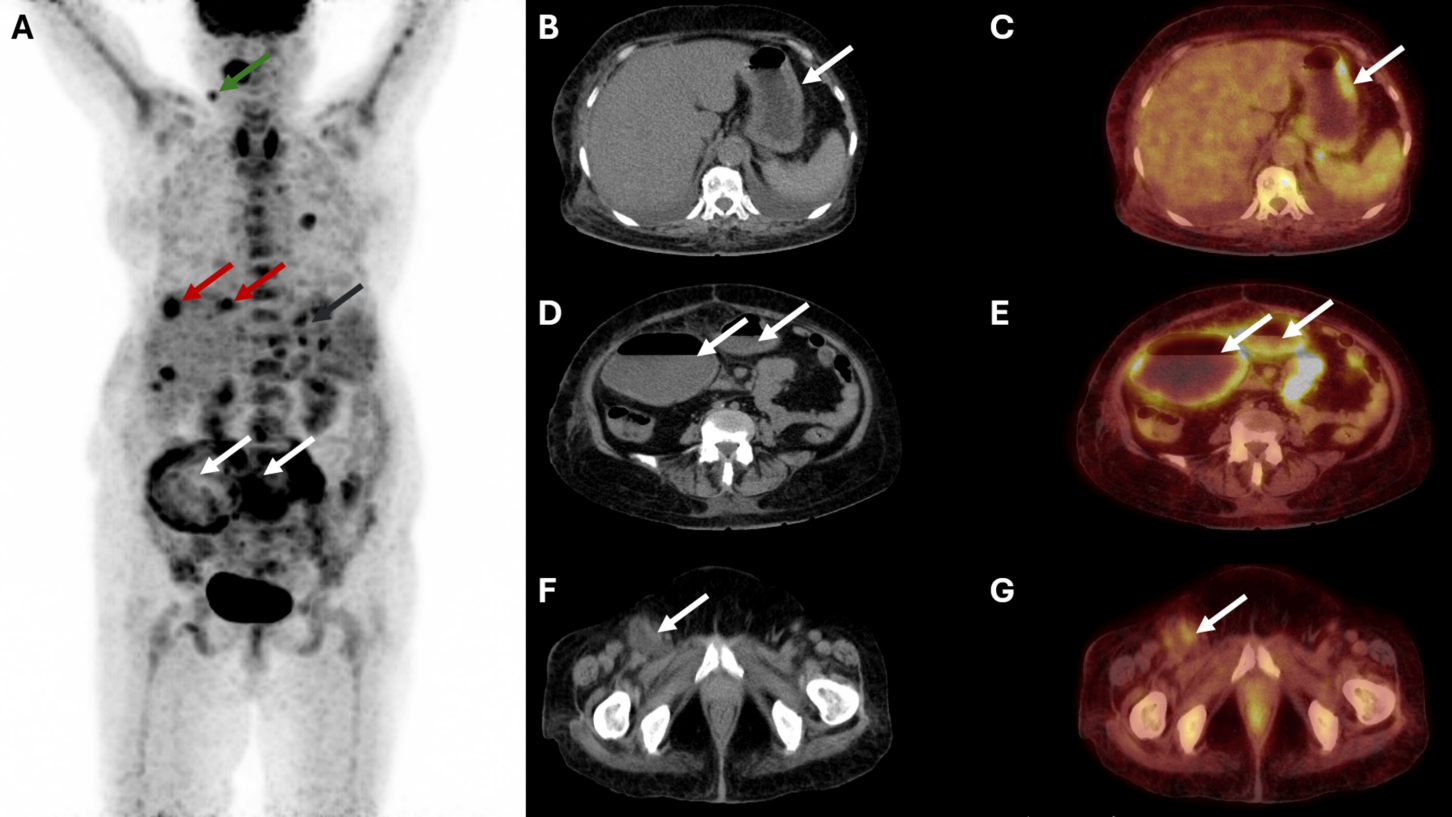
PET-CT images Maximum intensity projection (MIP) image (A) reveals a nodal deposit in the neck (green arrow). Deposits in the region of the liver (red arrows) and stomach (black arrow) are also noted. FDG uptake is noted in the region of the bowel loops (white arrows) in the abdomen. The CT image (B) and fused PET-CT image (C) reveal FDG-avid gastric wall thickening (white arrow). CT image (D) and fused PET-CT image (E) reveal FDG avid wall thickening involving dilated small bowel loops (white arrows). CT image (F) and fused PET-CT image (G) reveal a lymph node in the right inguinal region with evidence of necrosis (white arrow). FGD: fludeoxyglucose-18; PET-CT: positron emission tomography-computed tomography
